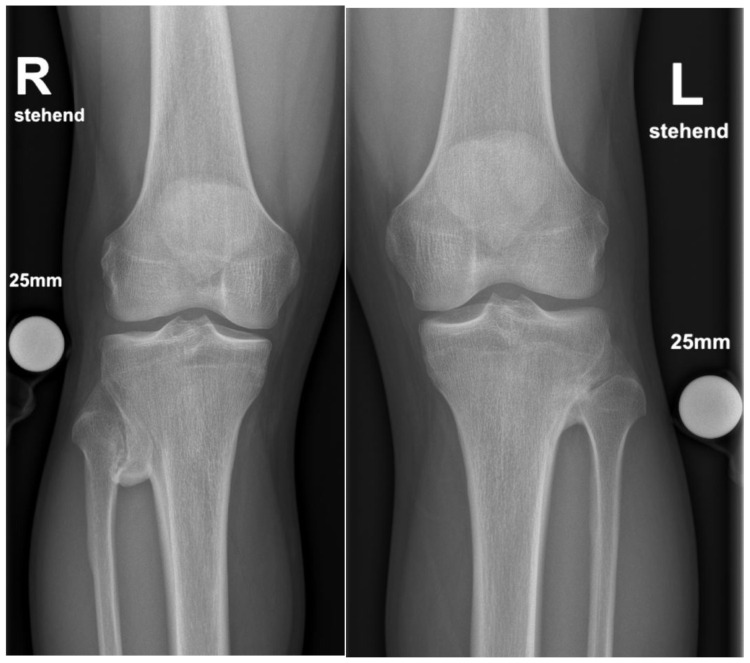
Plain radiograph in a.-p. view showing the bilateral synostosis.
